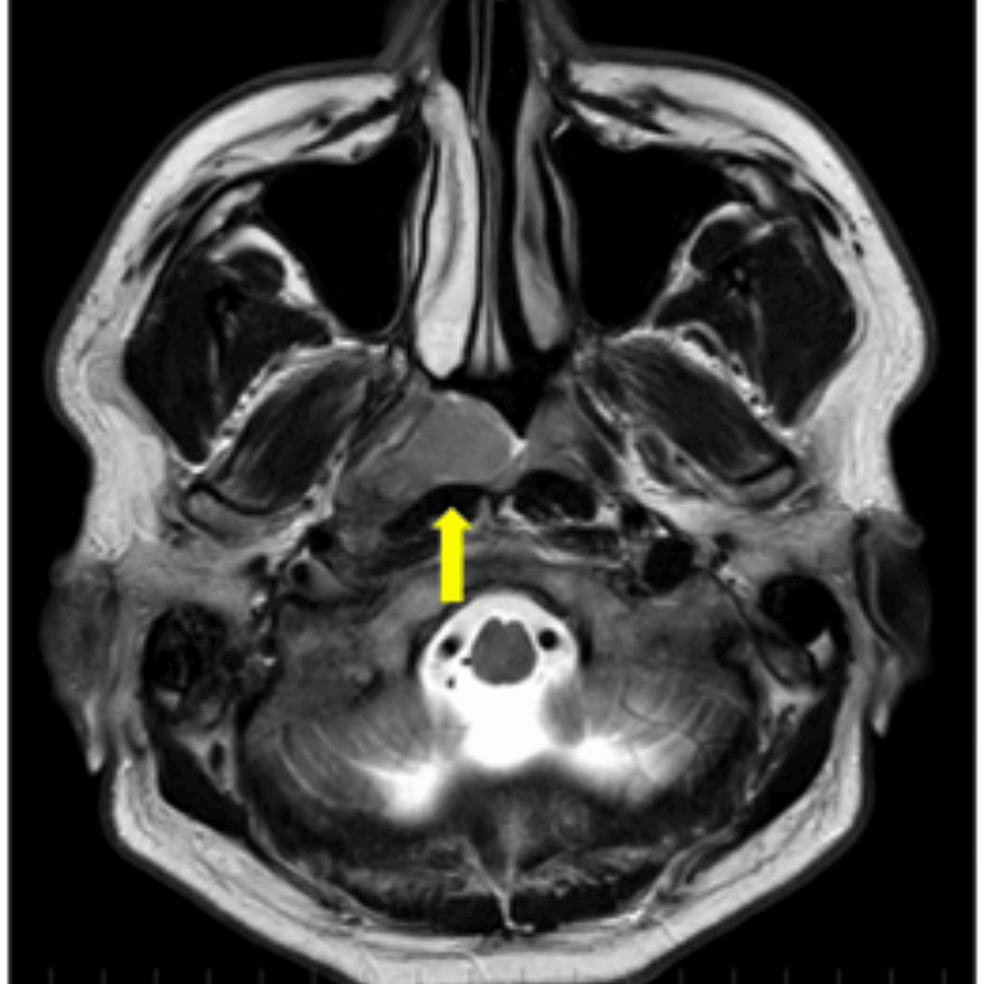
Magnetic resonance imaging at the initial diagnosis The tumor (arrow) extended into the parapharyngeal space and was diagnosed as cT2.
